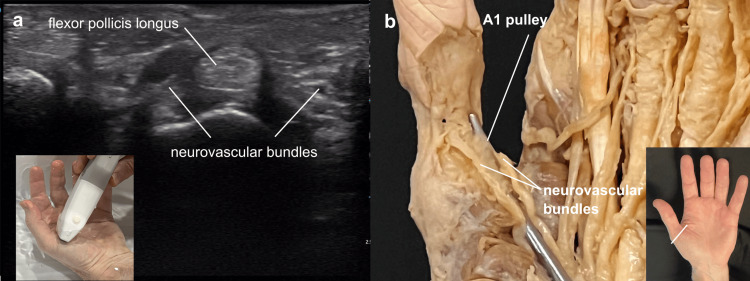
Stenosing tenosynovitis (trigger) thumb anatomy Stenosing tenosynovitis (trigger) thumb anatomy: (a) Short axis ultrasound view of the flexor pollicis longus of the left hand at the level of the A1 pulley, the probe marker oriented to the left to correlate with cadaver image, (b) cadaver view with an instrument passed through the A1 pulley of the thumb.
